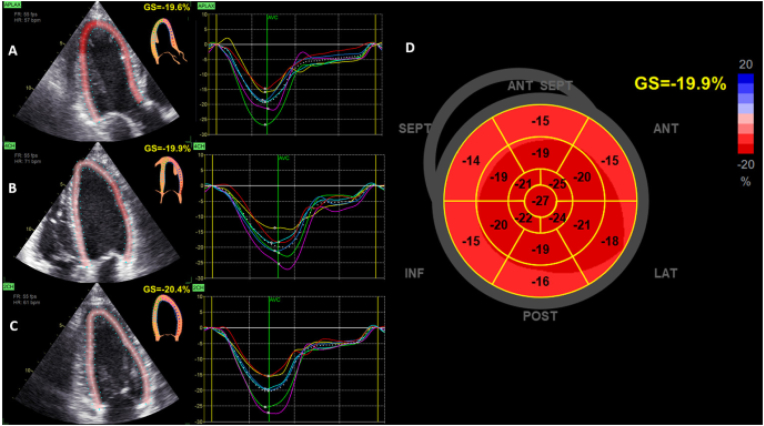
Left ventricular GLS assessment with two-dimensional speckle tracking echocardiography. The figure demonstrates analysis of left ventricular GLS from the three-chamber (A), four-chamber (4CH) (B) and two-chamber (2CH) (C) views, with the respective time to strain curves. The value of GLS is displayed in each view. (D) Polar map with the regional values and the GLS value calculated from the 17 segments which is within the normal value.
